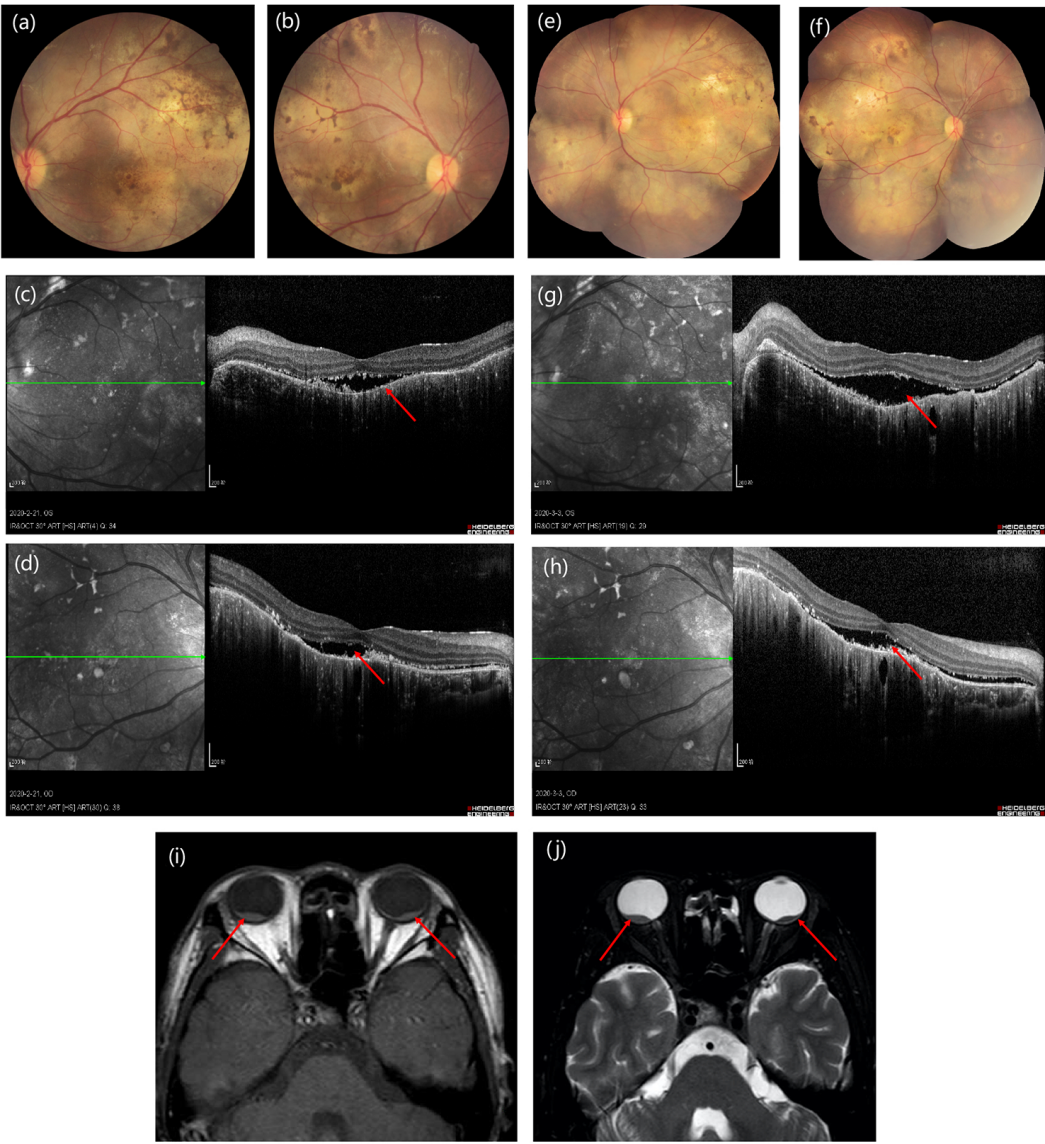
Time course of images of the presentation in fundus examination (A, B) and optical coherence tomography (C, D) at 3-week follow-up to fundus examination (E, F) and optical coherence tomography (G, H) at 6-week follow-up. (I, J) Eye MRI shows bilateral retinal detachment of optic nerve.
